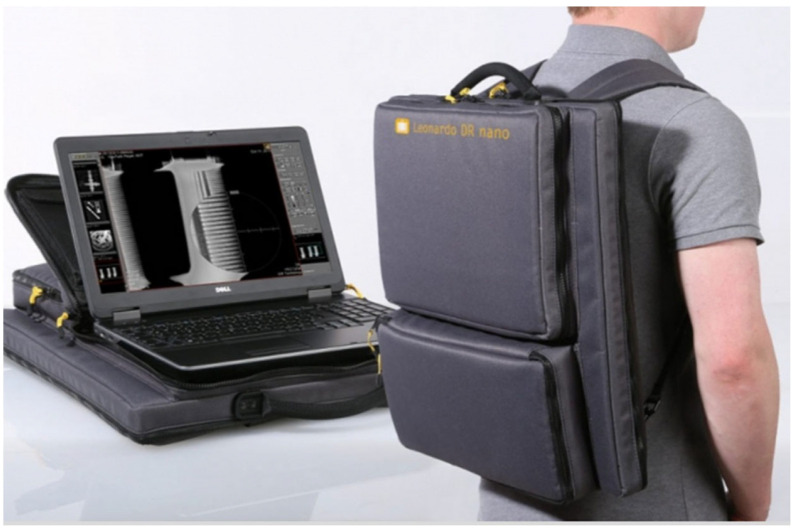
Image of a Leonardo nano mobile X-ray system.
